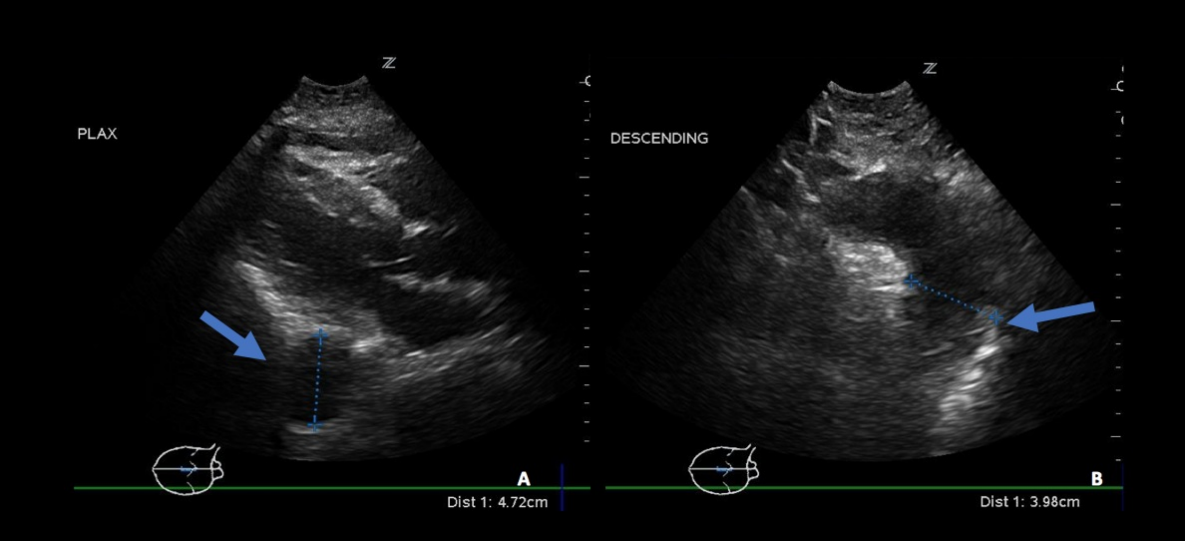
Thoracic aortic aneurysm. (A) Parasternal long axis (PLAX) imaging of the heart demonstrates an enlarged descending thoracic aorta. (B) Suprasternal Imaging demonstrates a grossly enlarged descending thoracic aortic root.
